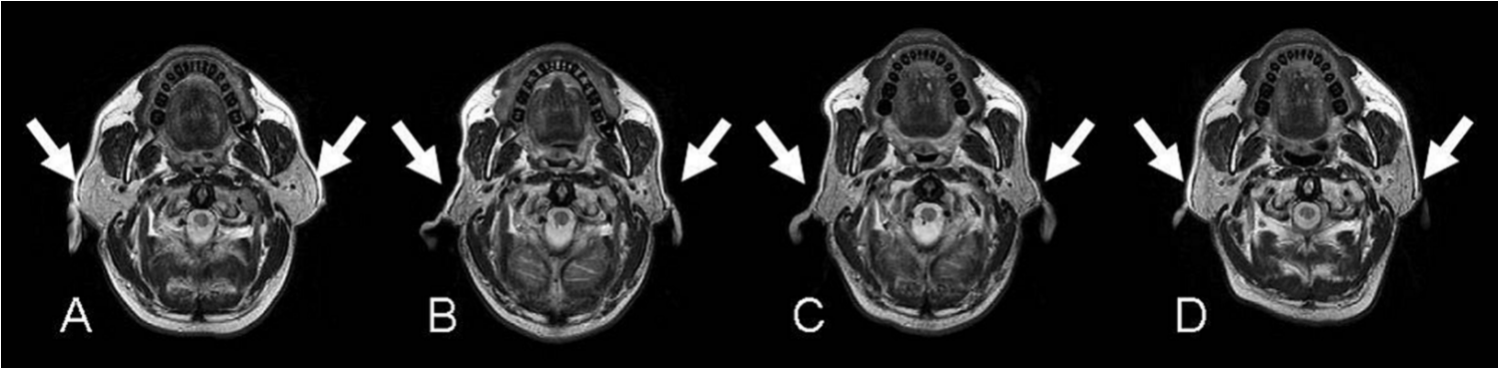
A 54-year-old patient with nasopharyngeal carcinoma with a radiation dose to the tumor, right parotid gland and left parotid gland of 72 Gy, 26.49 Gy and 24.75 Gy, respectively. Serial axial T2-weighted images of were obtained at MR stage 0 (3A; before radiotherapy), 1 (3B; 53 days), 2 (3C; 270 days) and 3 (3D; 435 days), respectively. The parotid glands (arrows) showed perceivable shrinkage at stage 1 followed by gradual restoration at stage 2 and stage 3.
